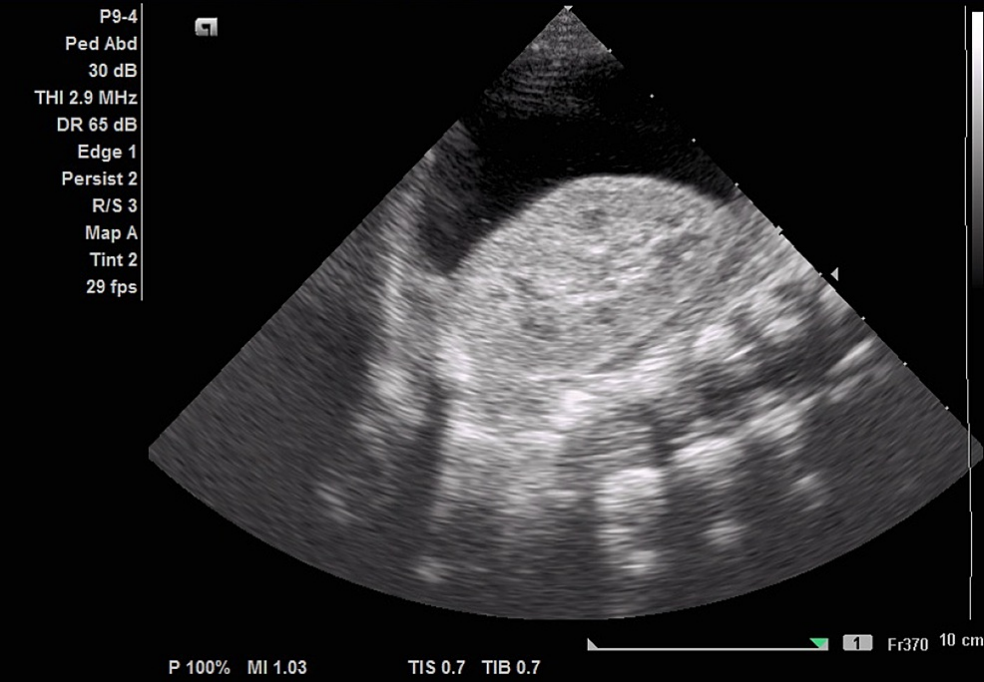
Ultrasound abdomen. Marked ascites with septations is seen.
